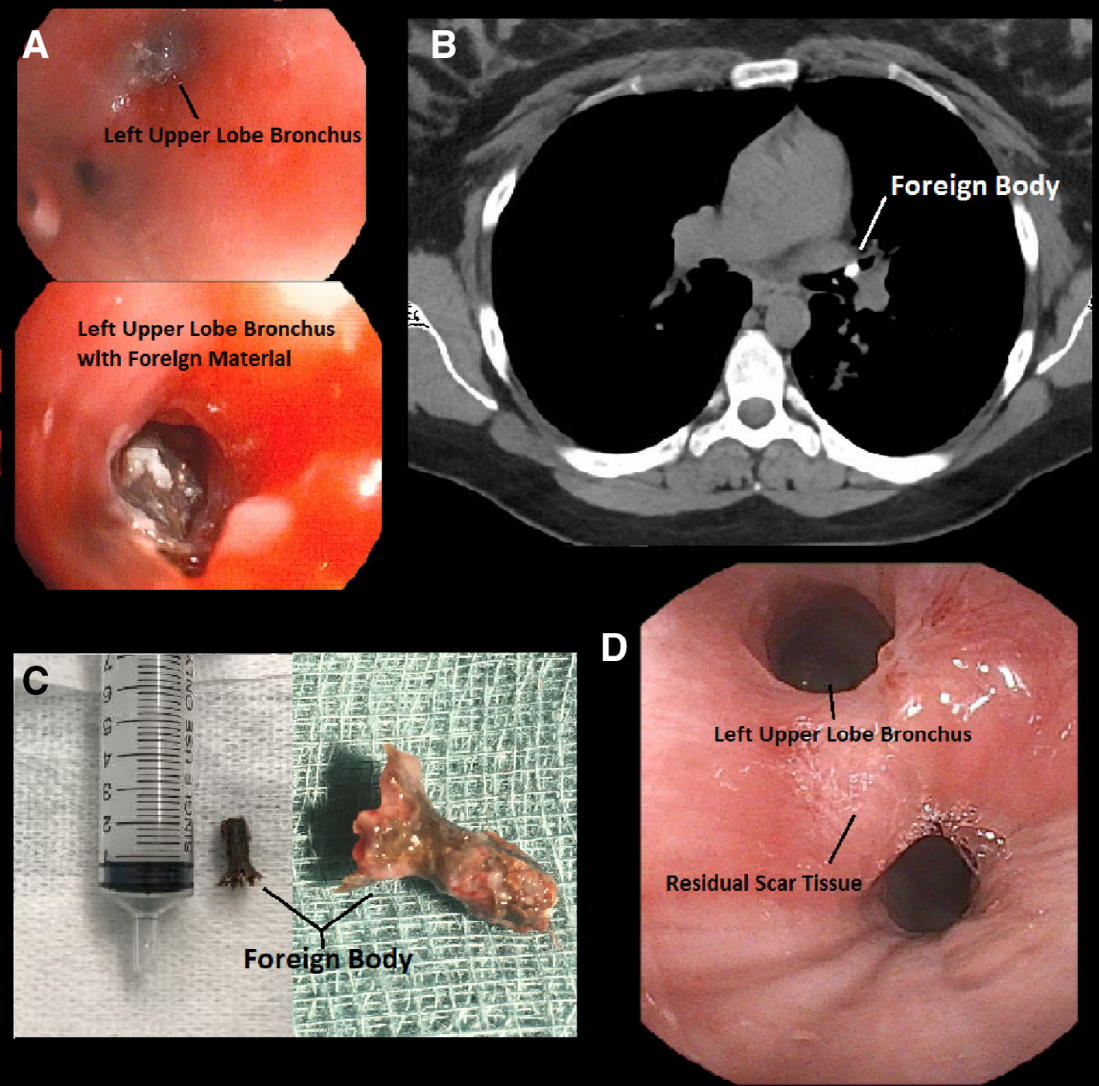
(A) Foreign body in the left upper lobe bronchus. (B) Foreign body identified on chest computed tomography. (C) Non‐organic foreign body. (D) Follow‐up bronchoscopy demonstrating patent left upper lobe bronchus.
